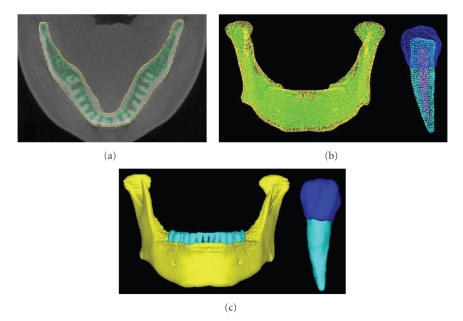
Geometry of 3D images and 3D models. (a) ROI of CT image, (b) 3D solid model, (c) 3D FE models.
